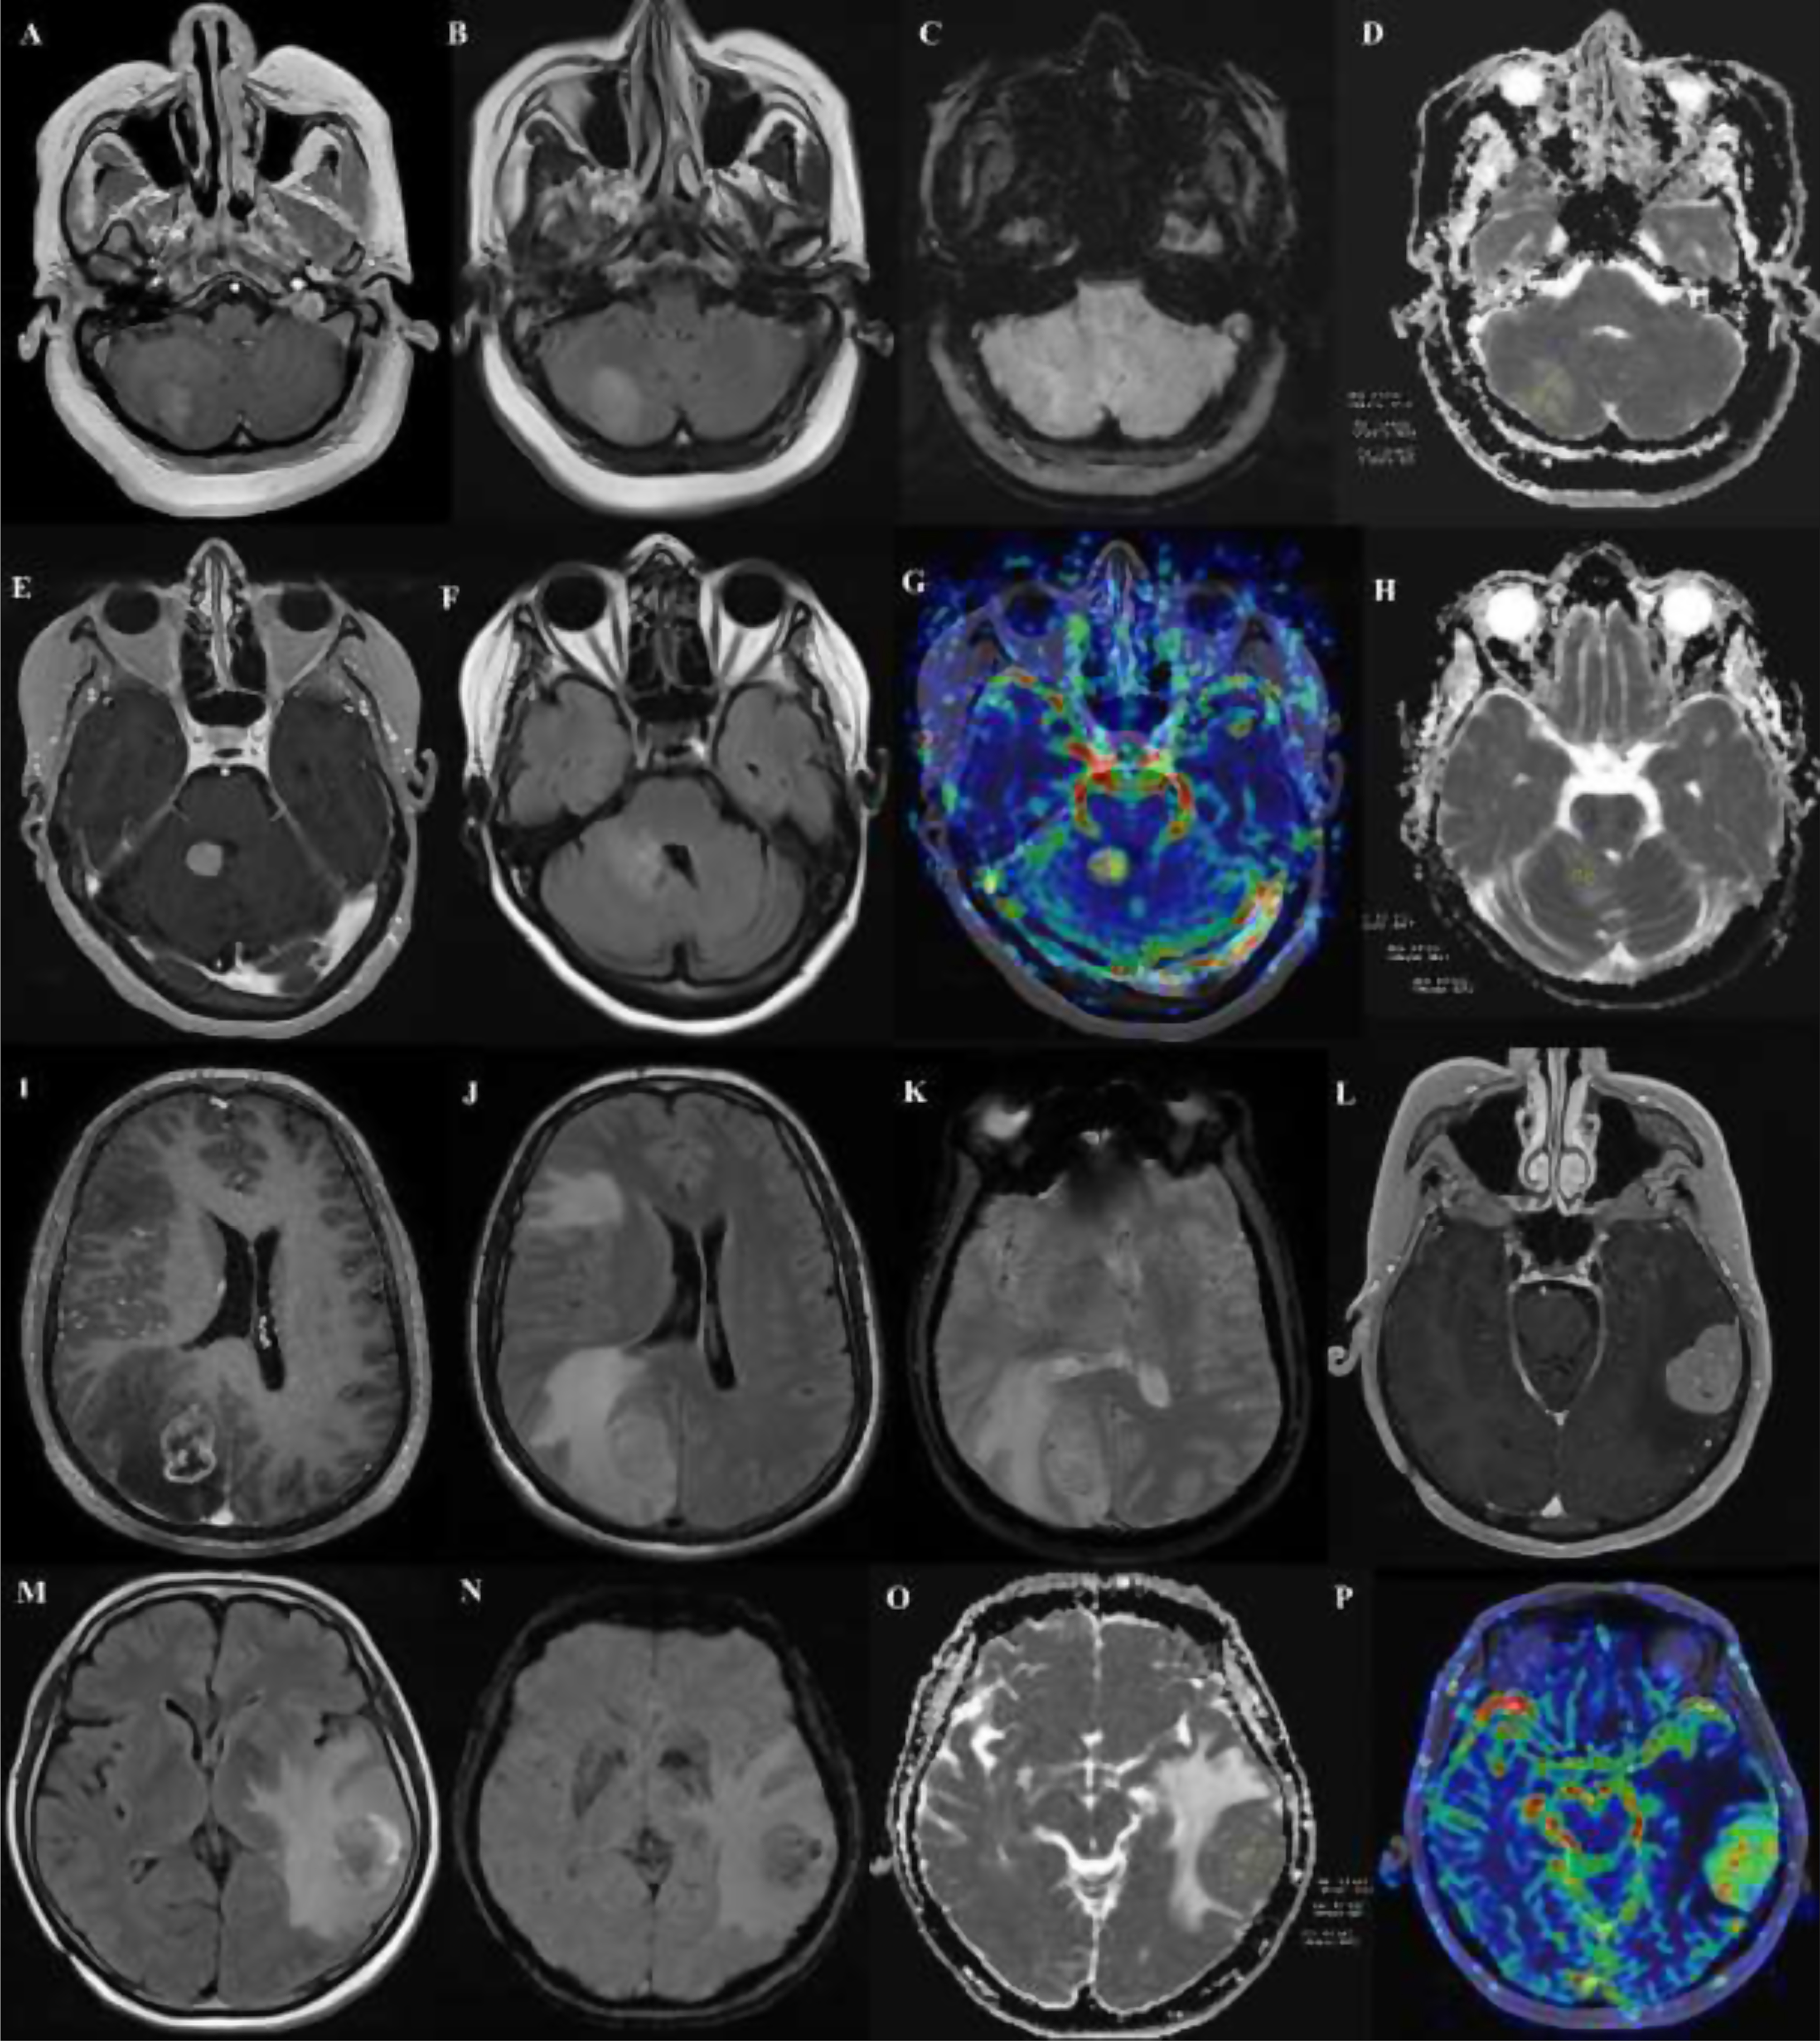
Representative multiparametric MRI findings of brain metastases from different primary tumors. (A–D) Breast cancer metastasis in the right cerebellar hemisphere. (A) Contrast-enhanced T1-weighted image demonstrates strong enhancement. (B) FLAIR image shows no significant peritumoral edema. (C) Susceptibility-weighted image reveals two punctate hypointense foci corresponding to an ITSS grade 1. (D) ADC map indicates a mean ADC value of 0.907 mm²/s. (E–H) Small cell lung cancer metastasis adjacent to the fourth ventricle. (E) Contrast-enhanced T1-weighted image shows an enhancing lesion. (F) FLAIR image shows minimal peritumoral edema. (G) Perfusion map demonstrates hyperperfusion, and (H) ADC map shows a mean ADC value of 0.829 mm²/s. (I–K) Non-small cell lung cancer metastasis with a central necrotic component. (I) Contrast-enhanced T1-weighted image shows irregular peripheral enhancement with central non-enhancing necrosis. (J) FLAIR image reveals extensive peritumoral edema. (K) Susceptibility-weighted image demonstrates two punctate hypointense signals (ITSS grade 1). (L–P) Malignant melanoma metastasis. (L) Contrast-enhanced T1-weighted image demonstrates vivid enhancement. (M) FLAIR image shows prominent peritumoral edema. (N) Susceptibility-weighted image reveals multiple hypointense foci (7–8 in total, ITSS grade 2). (O) ADC map shows a mean ADC value of 0.913 mm²/s. (P) Perfusion map demonstrates marked hyperperfusion
